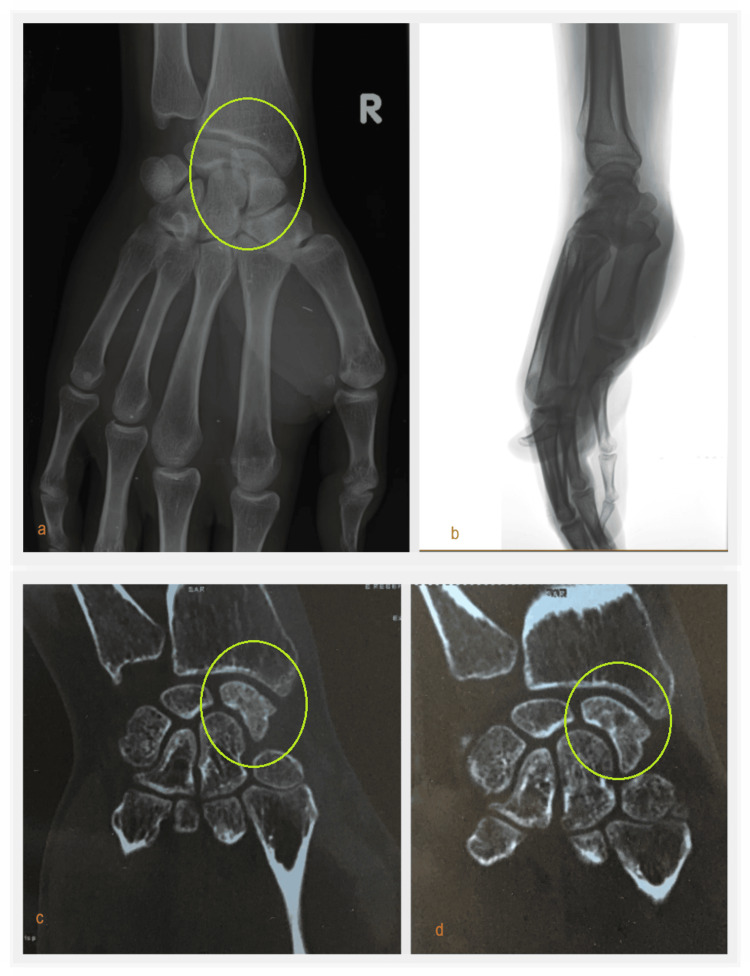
Imaging at follow-up demonstrating complete spontaneous union of the scaphoid, with CT confirmation of trabecular bridging and complete consolidation of the previous nonunion (a–d) Trabecular bridging and complete consolidation of the previous nonunion (green circle).
